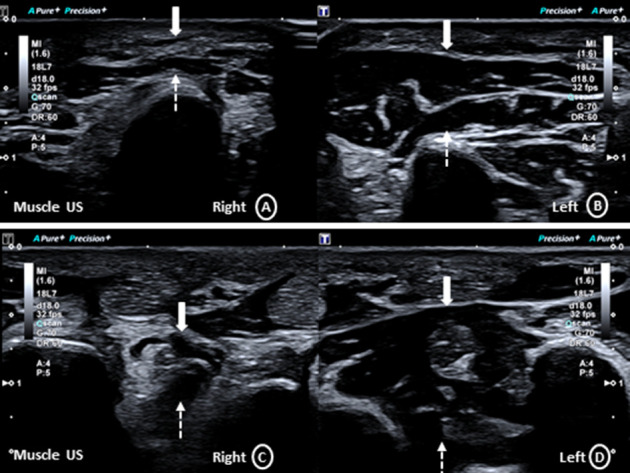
Axial muscle ultrasound of the right (A) and left (B) hypothenar as well as right (C) and left (D) interdigital muscles, with marked hyperechogenic atrophy of the abductor digiti minimi and dorsal interosseus muscles on the right side.
